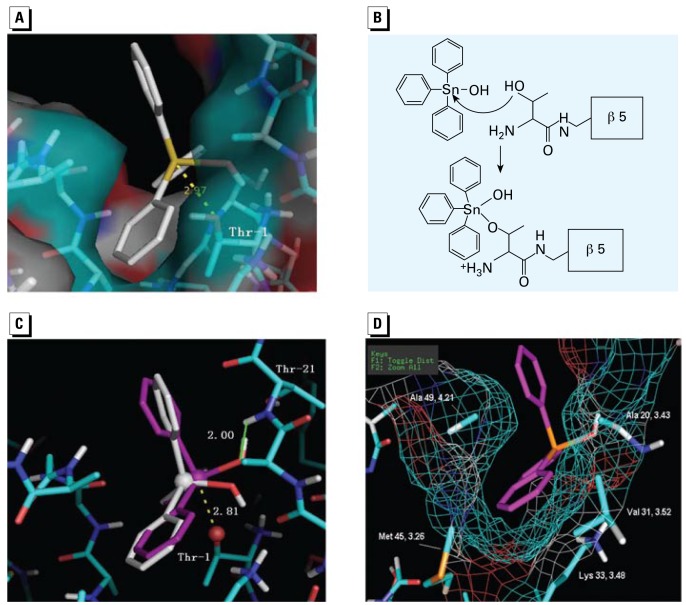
Docking studies for the interaction of TPT and the proteasome β5 subunit. (*A*) The docking model of TPT-OH with a tetrahedral conformation in the proteasomal β5 CT site; TPT-OH is represented by a stick structure. (*B*) A hypothetical mechanism for the interaction between TPT-OH and the β5 Thr-1 residue. (*C*) Comparison of TPT-OH with tetrahedral conformation (white) and trigonal bipyramidal conformation (pink) in the β5 site. The three phenyl rings, one OH legend, and O^γ^ on Thr-1 form the trigonal bi pyramidal conformation; the Sn on TPT and O^γ^ of Thr-1 are shown as spheres. (*D*) The hydrophobic interaction between the phenyl ring of TPT and the hydrophobic side chains in the S1 pocket. The mesh represents the surface of the S1 pocket.
